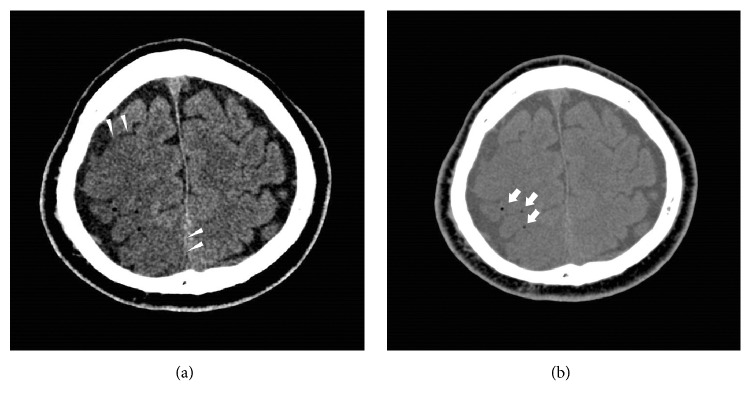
The same slice of head CT on the day of the onset of CAAE. The arrowheads show the obscureness of the corticomedullary junction. The arrows show the embolization of air bubbles. (a) Displayed with normal brain window settings (window width, 80 Hounsfield Units (HU); window level, 40 HU). (b) Displayed with mediastinal window settings (window width, 250 HU; window level, 40 HU).
